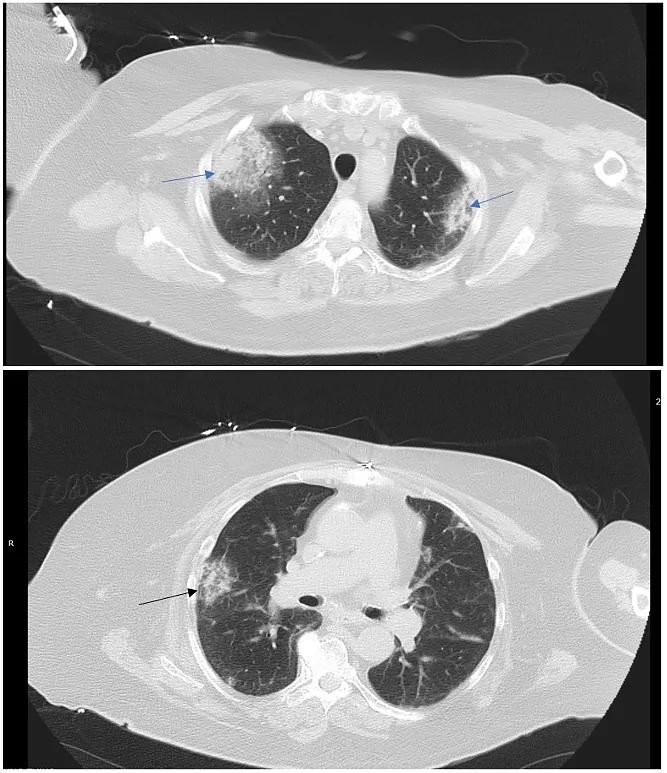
CT Chest without contrast (Before discontinuation of Daptomycin) showing new consolidative changes in both upper lobes, mainly the right upper lobe (blue arrows), with ground glass opacities extending to the right middle lobe (black arrow).
